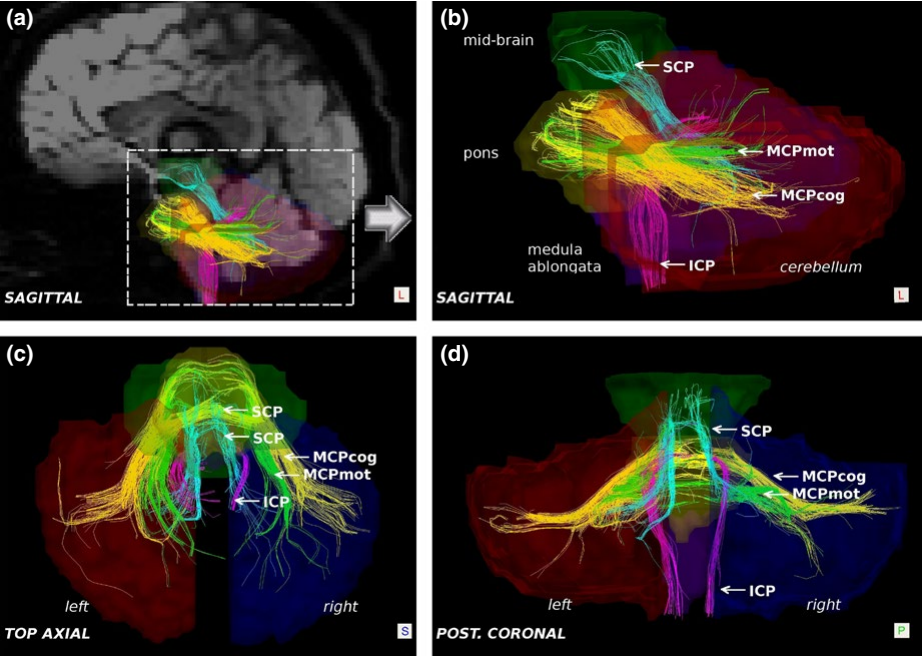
Example tractography representation of cerebellar peduncle pathways in a young adult (21Y). (a) Left sagittal whole‐brain view showing tractography results superimposed on a medial‐sagittal DWI image. Also indicated in this panel is the region of focus displayed in other panels (dashed line rectangle). (b) Left sagittal view focused on cerebellar‐brainstem region. (c) Top axial view. (d) Posterior coronal view. Anatomical structures shown in transparency as labeled. Tracts are color‐coded as: superior cerebellar peduncle pathway (SCP) in light blue, inferior cerebellar peduncle pathway (ICP) in pink, MCPcog (higher cognitive portion of MCP) in yellow, and MCPmot (motor portion of MCP) in green. See text for details
